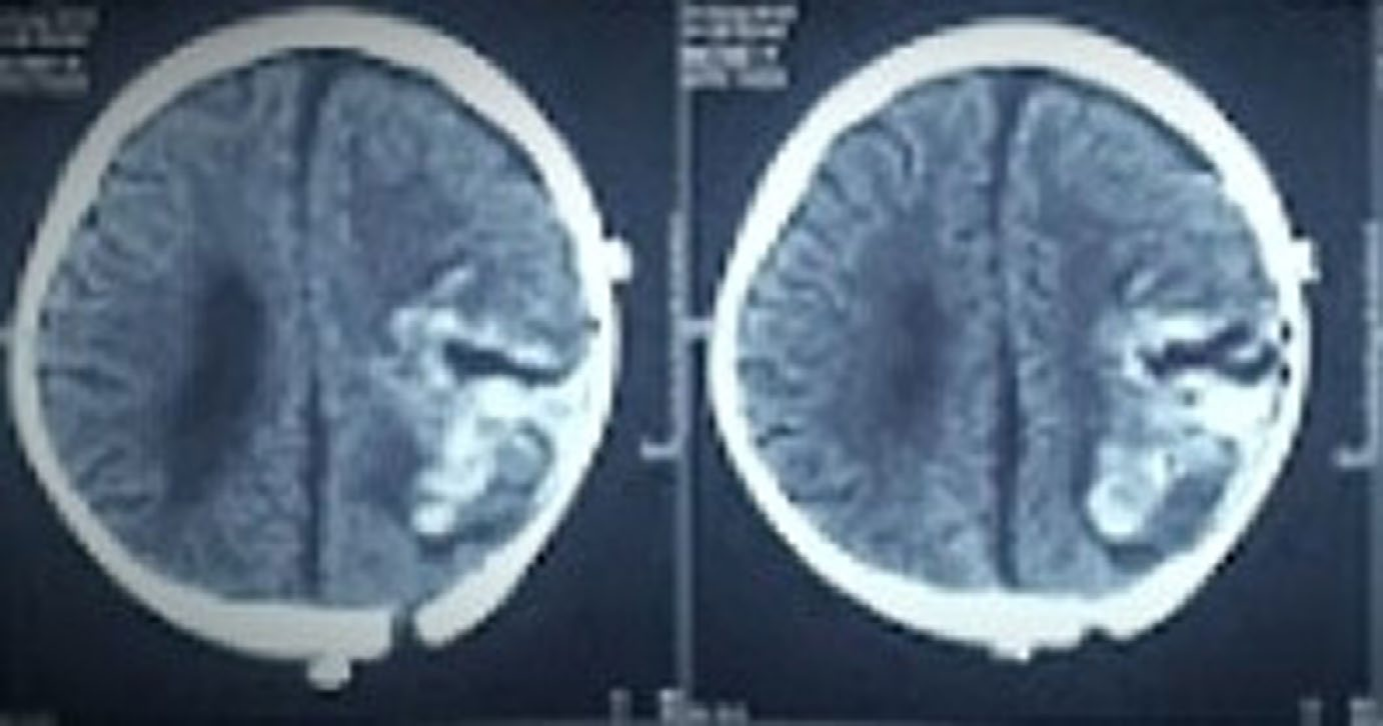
Post‐op CT scan showing hematoma at the operative site
